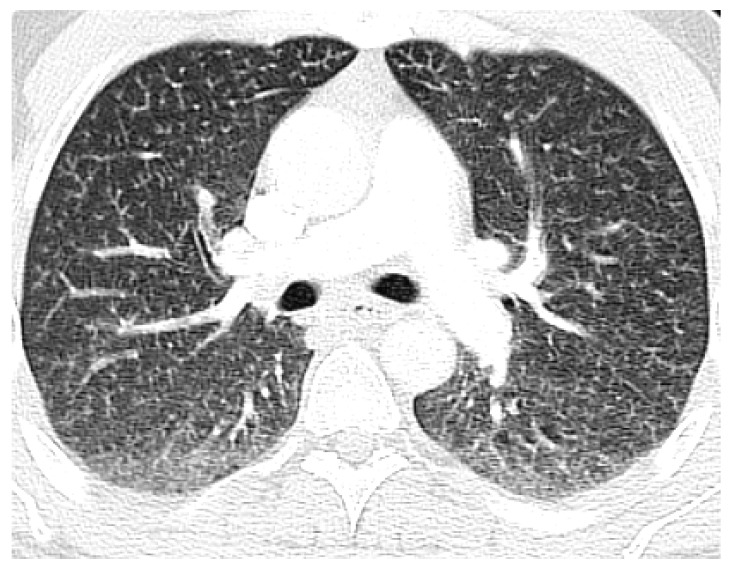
Axial computer tomography angiography (CTA) at the level pulmonary arteries showing extensive military densities in the bilateral lung fields with no evidence of embolism.
